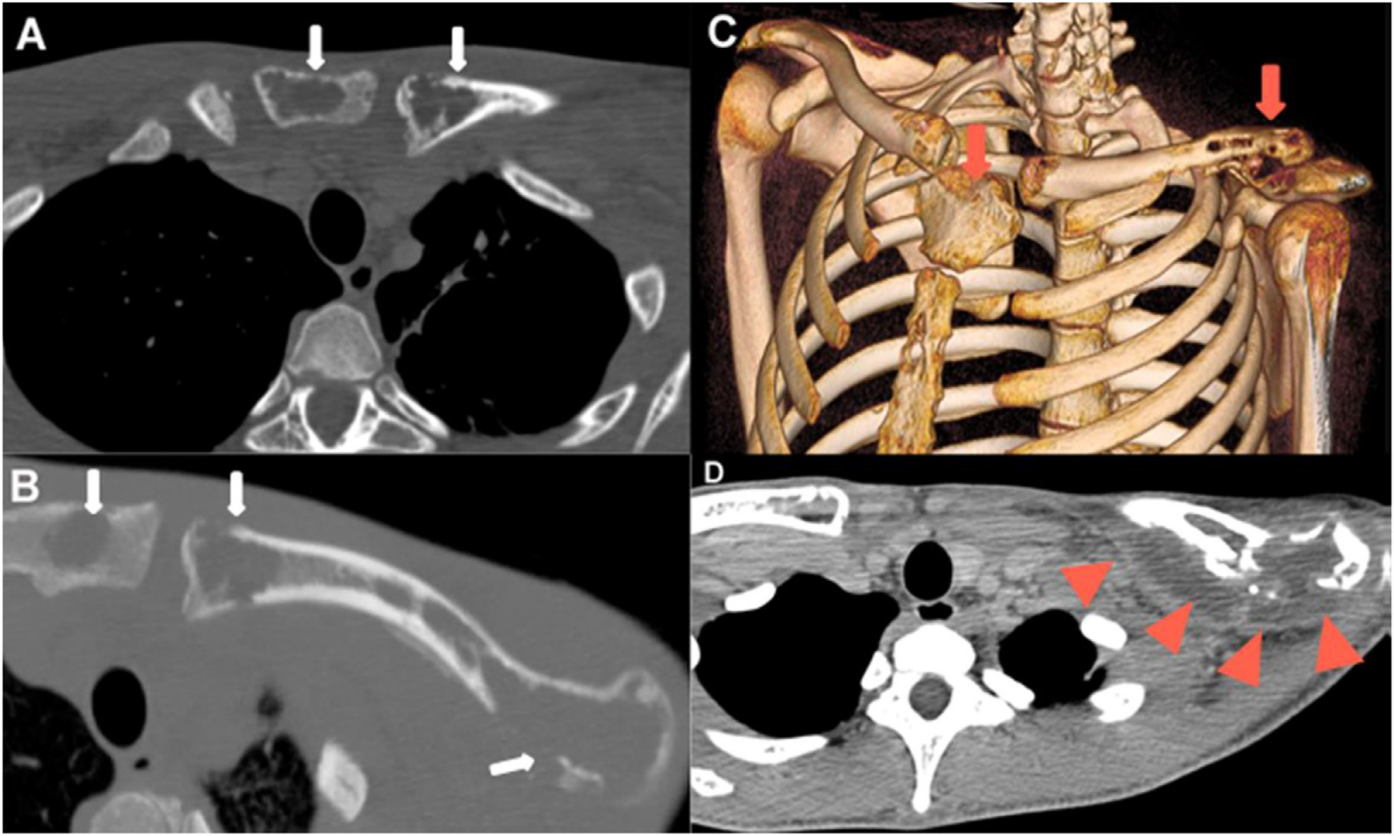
(A‒B) CT images obtained in the axial plane and bone window showing lytic lesion signs with cortical destruction of the left clavicle and manubrium and no periosteal reaction (white arrows). (C) Volume-rendered 3D image also demonstrating multiple osteolytic lesions with cortical rupture of the manubrium, sternum, and left clavicle (red arrows). (D) CT image obtained in the axial plane and soft-tissue window depicting fluid collection around the bony destruction of the left clavicle (red arrowheads).
